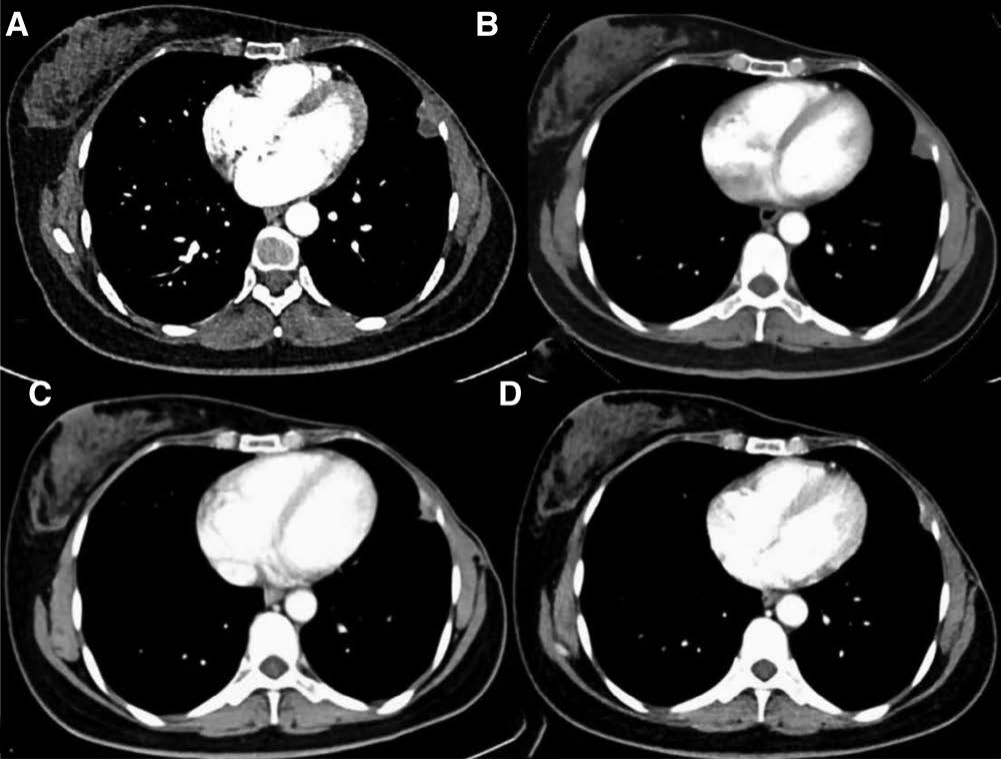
Serial contrast-enhanced axial CT images of the chest wall lesion. (A) Baseline (pretreatment), (B) 1-month posttreatment, (C) 3 months posttreatment, (D) 6 months posttreatment.
